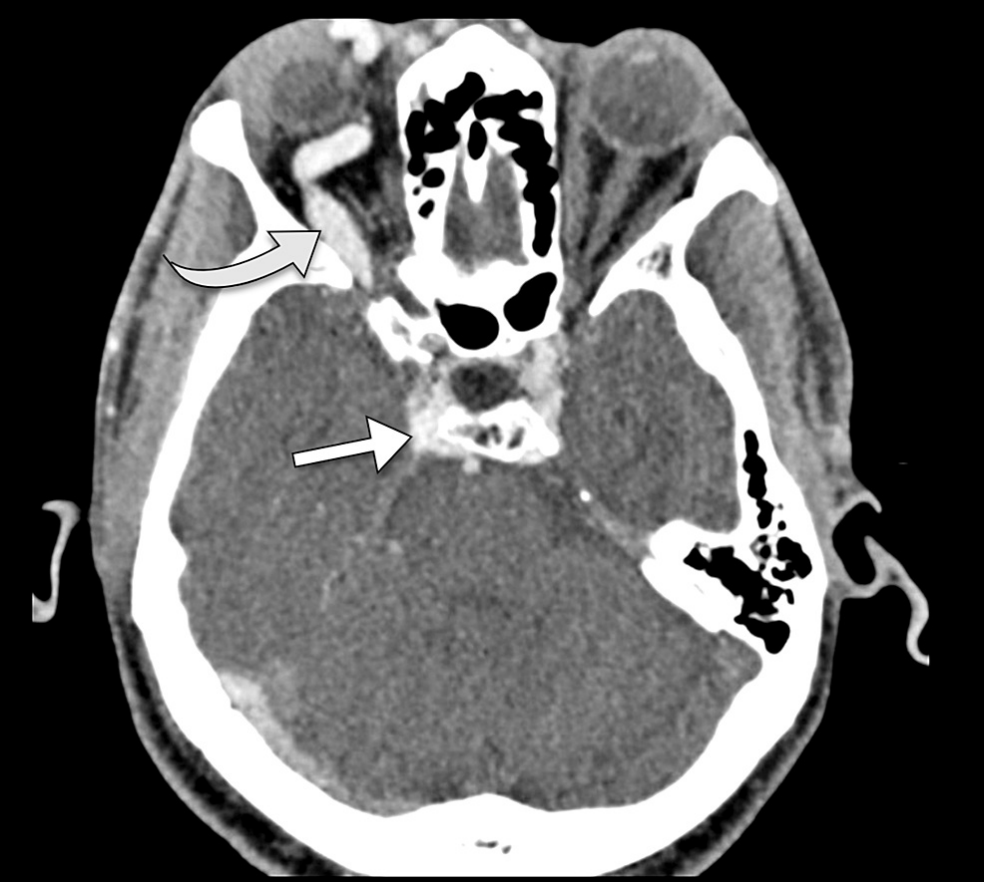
A contrast-enhanced axial CT image depicting a distended right cavernous sinus (arrow), accompanied by an enlarged right superior ophthalmic vein (curved arrow), is indicative of a right carotid-cavernous fistula.
